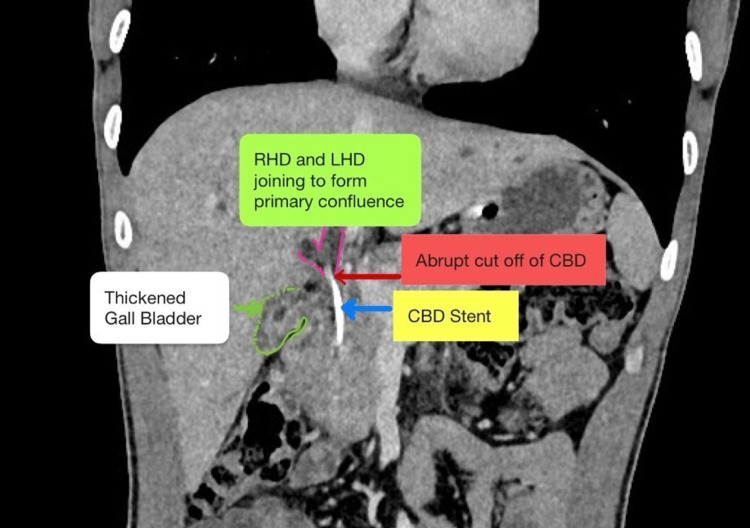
CT scan showing abrupt cut-off at proximal CBD with stent in situ and thickened GB. Coronal section of CT scan. GB: gall bladder; CBD: common bile duct; RHD: right hepatic duct; LHD: left hepatic duct.
